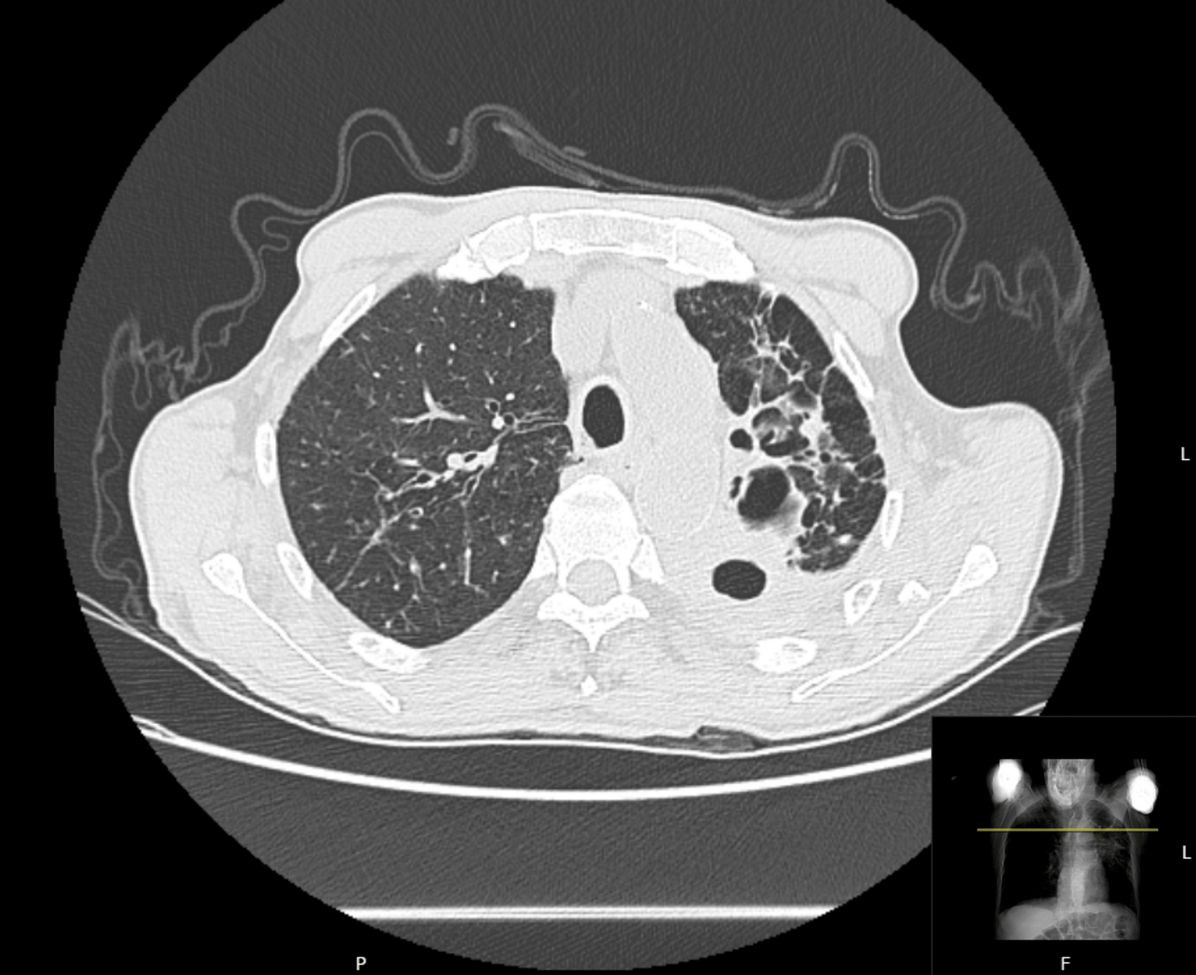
Preoperative chest computed tomography - axial slice with scanogram Preoperative chest computed tomography at the level of the upper lobes revealed severe architectural distortion of the apical region of the left lung. In the lower right corner, the scanogram (scout view) displays a coronal reference image, with a horizontal yellow line indicating the anatomical level of the axial slice shown.
